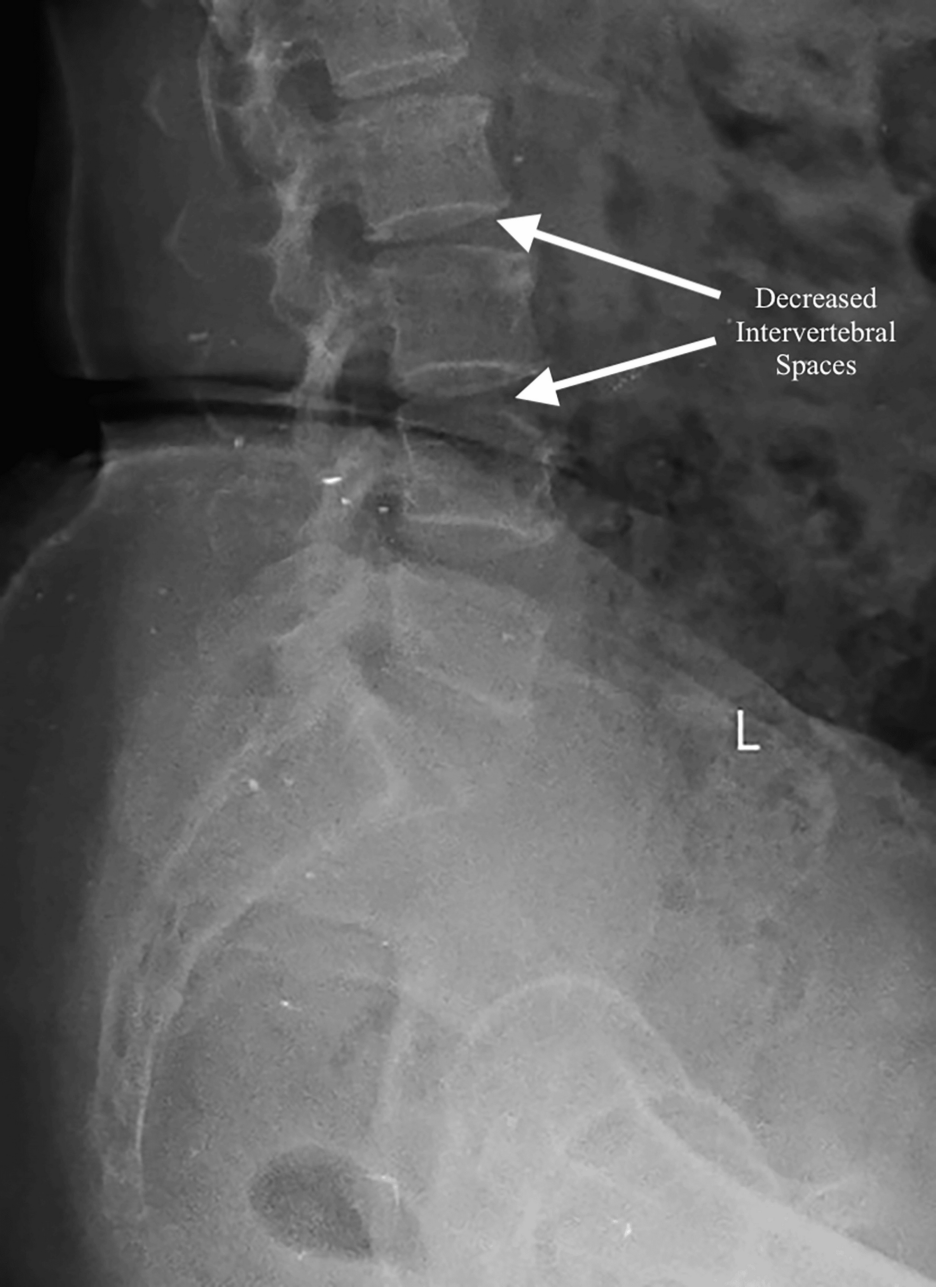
Xray of the lumbar spine showing decreased intervertebral spaces
